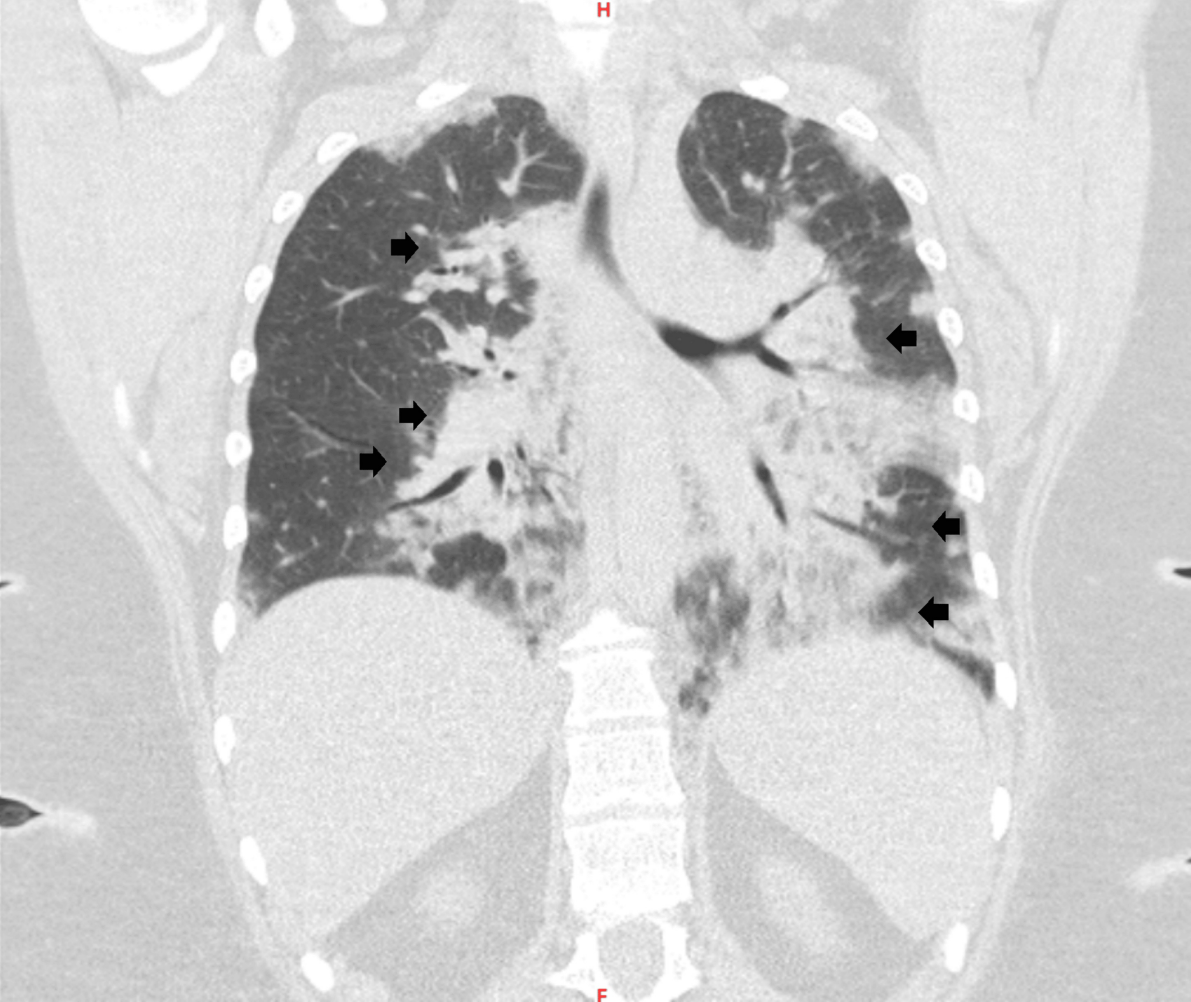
Computed tomography coronal slice: air bronchogram with central and peripheral involvement, with approximately 40% of the lung parenchyma compromised. Black arrows: multiple alveolar opacities are observed in the lung parenchyma.
